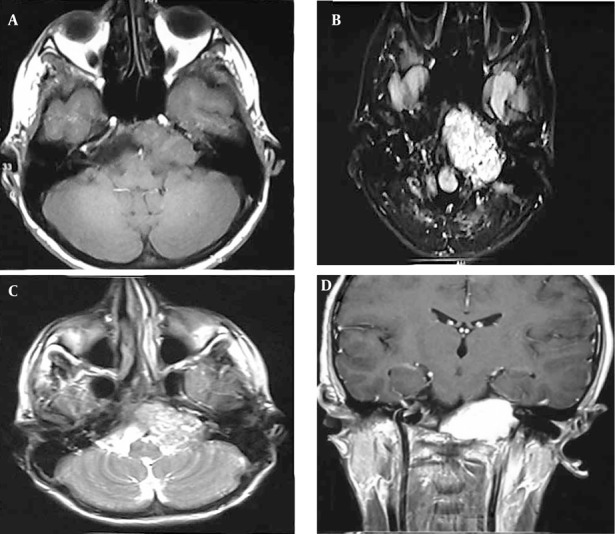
(A-D) Axial and coronal MRI with and without gadolinium: An iso (T1) to heterogeneous iso to high signal (T2, Flair) strongly enhancing lobulated well-defined mass in the left jugular foramen.
